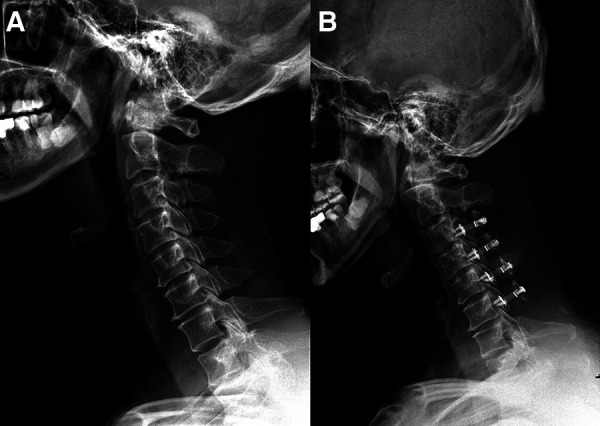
A 58-year-old male patient treated with C3–C6 laminoplasty. (A) Preoperative lateral cervical radiograph (CSVA = 37.6 mm, T1S = 28°). (B) Lateral cervical radiograph at final follow-up (17 months after surgery, CSVA = 41.2 mm).
